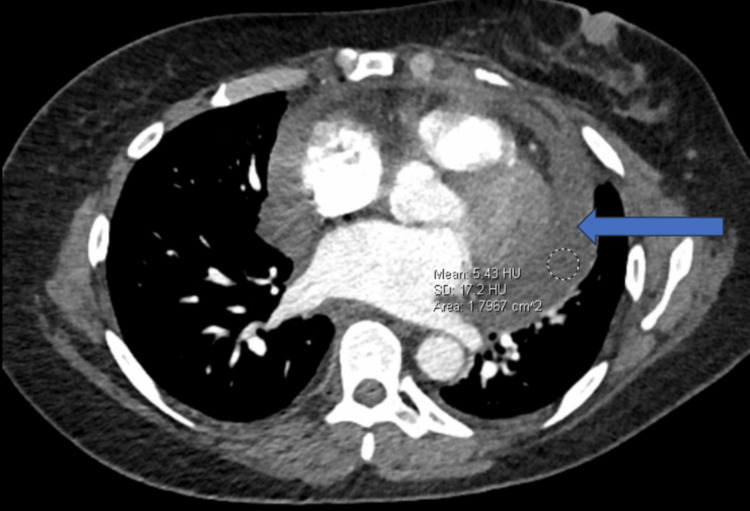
CT of the chest demonstrating pericardial effusion (blue arrow).
